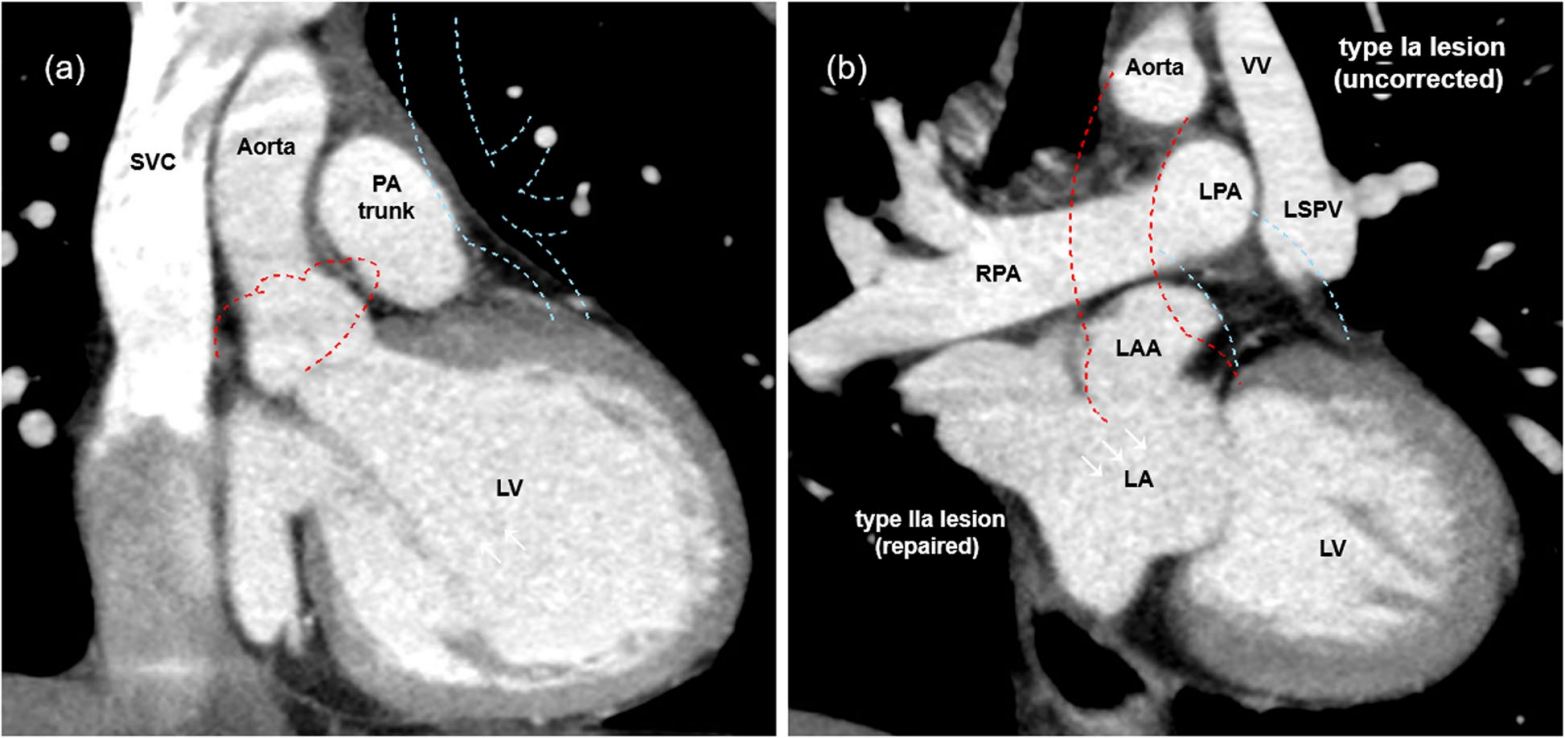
Preoperative computed tomography. a, b Coronal section of the preoperative computed tomography image reveals that the LAA (red dotted line in (a)) is located behind the aorta (red dotted line in (b)) and the LAA and LSPV (blue dotted line in (a)) are spatially separated by the PA trunk (blue dotted line in (b)). LA, left atrium; LAA, left atrial appendage; LPA, left pulmonary artery; LSPV, left superior pulmonary vein; LV, left ventricle; PA, pulmonary artery; RPA, right pulmonary artery; SVC, superior vena cava; VV, vertical vein
